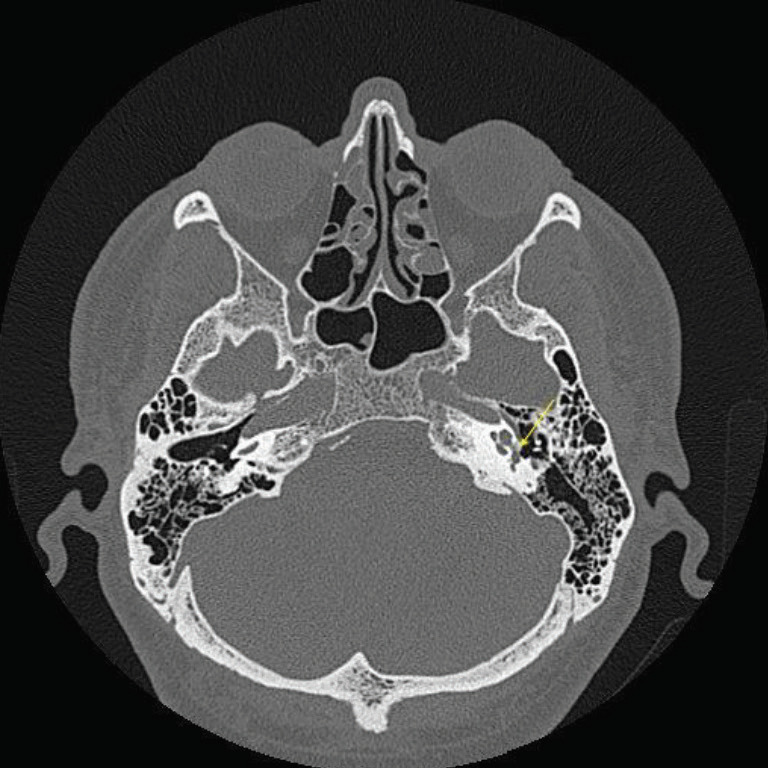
CT scan (axial plane) of patient (S5) shows left sided fenestral otosclerosis (yellow arrow: left sided fenestral involvement).
